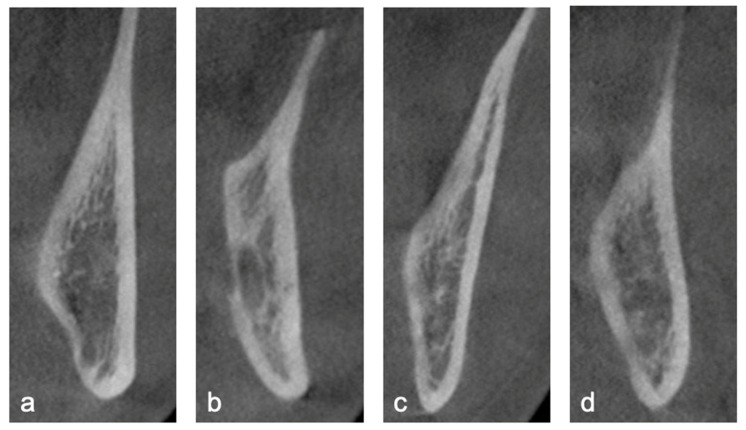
Lingual plate morphology at the level of root apex identified on sagittal CBCT section. (a) Type U, undercut on the lingual side, (b) Type P, parallel to the buccal plate, (c) Type S, slanted with buccolingual width reduced on the lingual side, (d) Type R, round shape on the lingual side.
